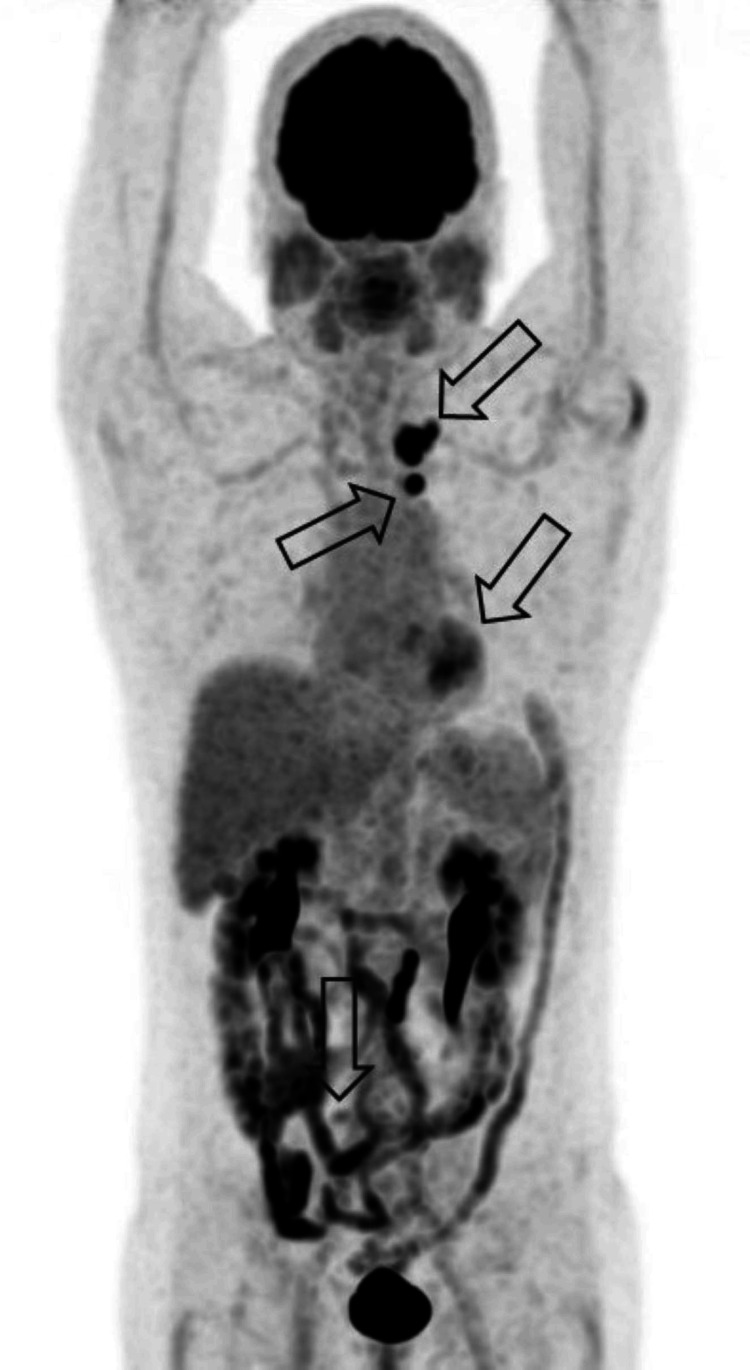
18-FDG PET scan performed before the treatment showed recurrence in the left retroclavicular lymph, left upper mediastinum, para-aortic, and lumbo-aortic nodes 18-FDG: fludeoxyglucose F18; PET: positron emission tomography
